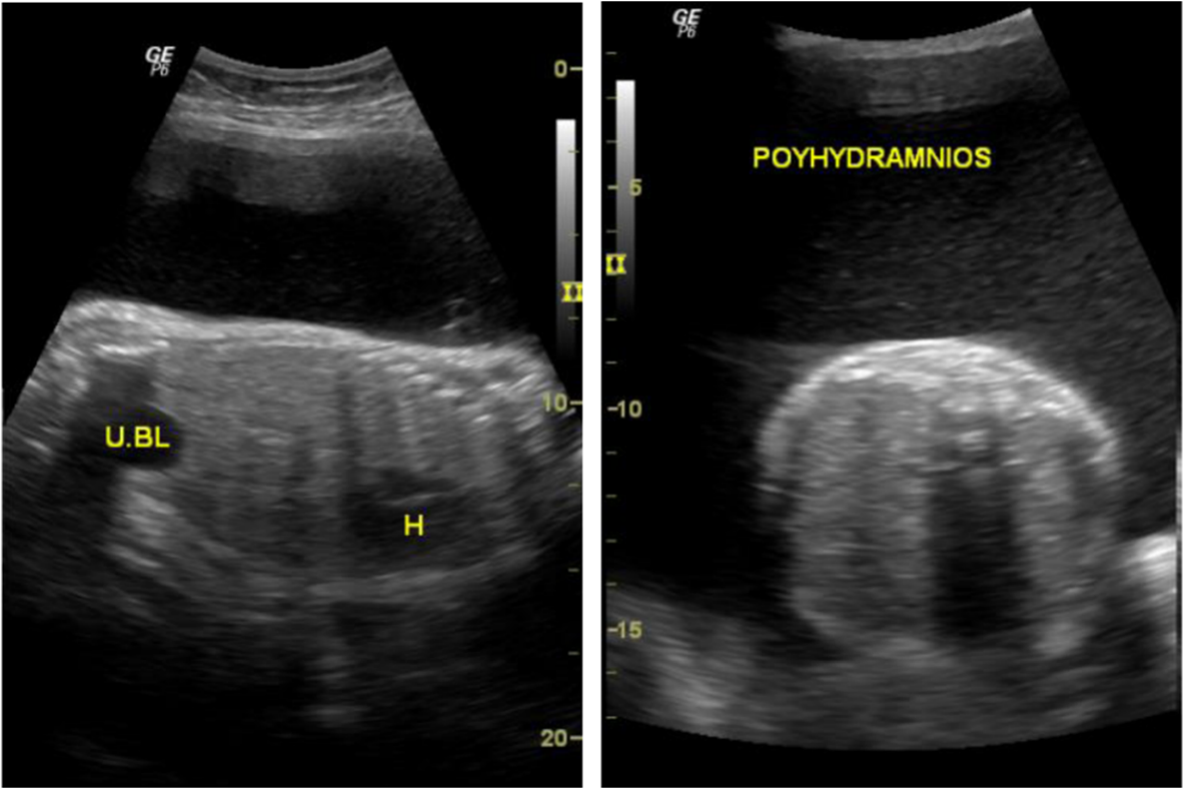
The above images were obtained from a mother age range 30-34 years Gravida 4 on routine sonography to assess fetal wellbeing. She had had previous normal pregnancies and was not on folate supplementation. The scans showed a ventricular atrial diameter of 20.1 mm and a diagnosis of ventriculomegaly was made
